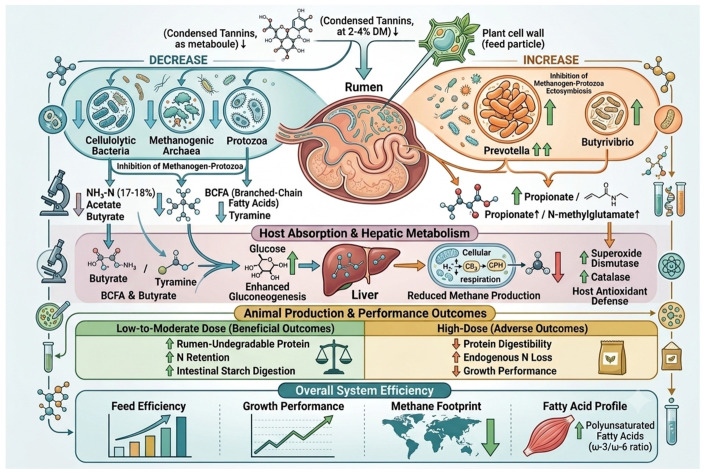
Mechanistic network of condensed tannins in ruminant nutrition and metabolism. The figure presents the dose-dependent effects of condensed tannins (2–4% DM) on rumen microbiota, metabolite shifts, host metabolism, and production/environmental outcomes in herbivores. Moderate tannin inclusion modulates microbial community and fermentation to improve nitrogen efficiency and reduce methane emissions, while high doses induce metabolic disorders. Note: ↑ = Increase, ↓ = Decrease. Blue: Beneficial effects of low-moderate CT (2–4% DM). Orange: Promoted microbes and metabolites. Yellow: Adverse effects of high-dose CT. Abbreviations: ANF: Anti-Nutritional Factor; DM: Dry Matter; BCFA: Branched-Chain Fatty Acids; NH_3_-N: Ammonia Nitrogen; N: nitrogen.
